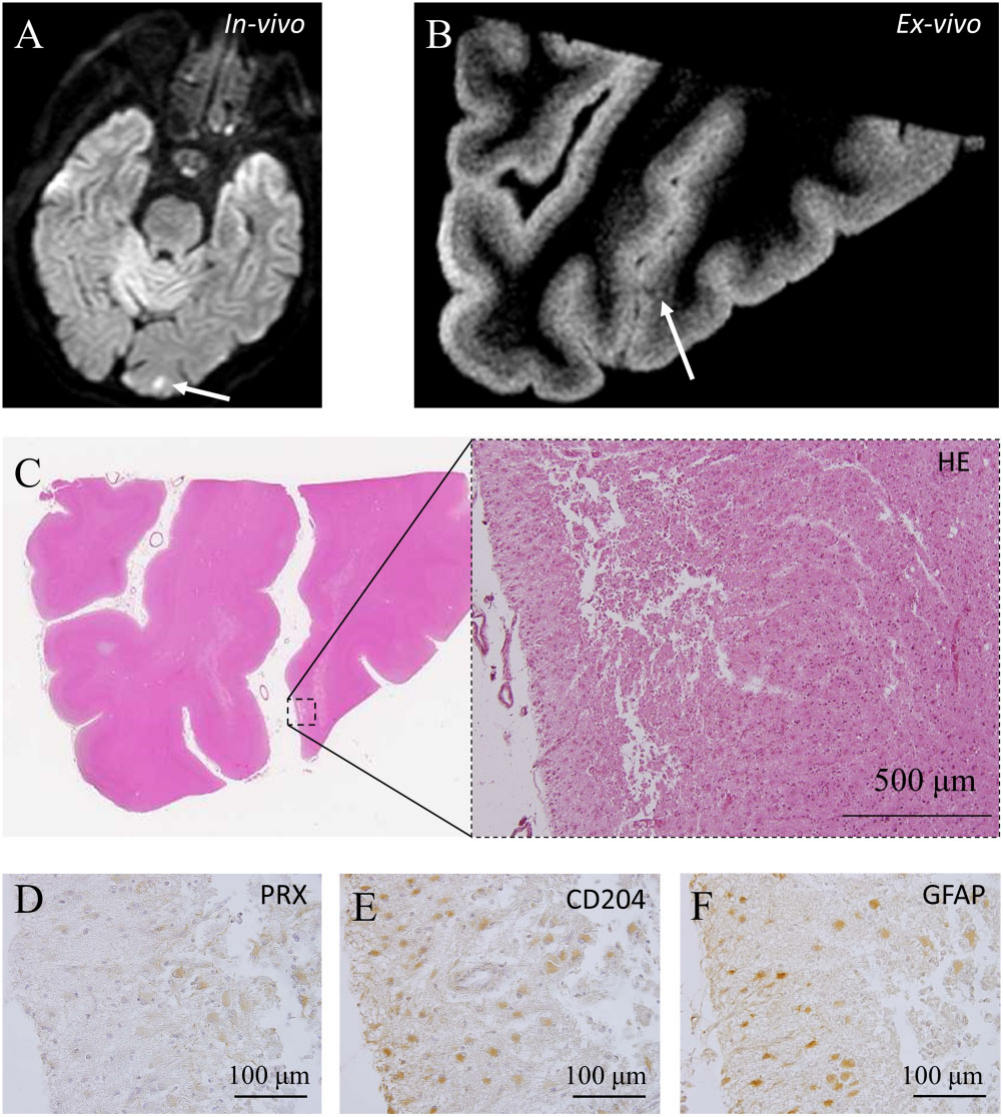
An acute cortical microembolism in Case 4. An acute cortical microembolism was observed on *in vivo* MRI (A). A hypointense lesion was observed on the T_1_-weighted image of *ex vivo* micro-MRI (B). The radiologically suspected ischaemic lesion was neuropathologically shown to be a microinfarction that extended to the subcortical area (C). The microinfarction was positive for peroxiredoxin (D), CD 204 (E) and GFAP (F), and suggestive of inflammation and a neuroprotective reaction. CD, cluster of differentiation; GFAP, glial fibrillary acidic protein.
